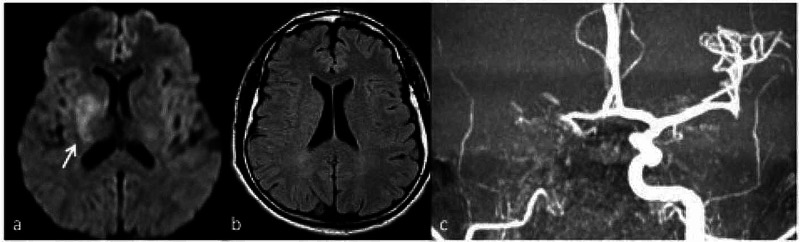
Brain magnetic resonance imaging a) Diffusion-weighted imaging, axial plan, showed a signal restriction in the right region of basal ganglia (arrow) without corresponding hyperintensity in T2w fluid attenuation inversional recovery (FLAIR) (b). The findings are consistent with acute stroke, confirmed by maximum intensity projection in coronal plan (c) of time of flight sequence showed a right tandem occlusion of internal carotid (arrowhead) and tract M1 of the middle cerebral artery (arrow).
